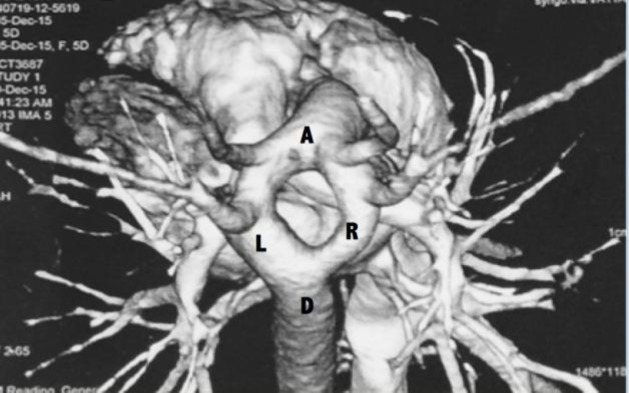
Computed tomogram (3D reconstruction) of the arch of the aorta showing a complete vascular ring. A, ascending aorta; D, descending aorta; L/R, left and right arch
